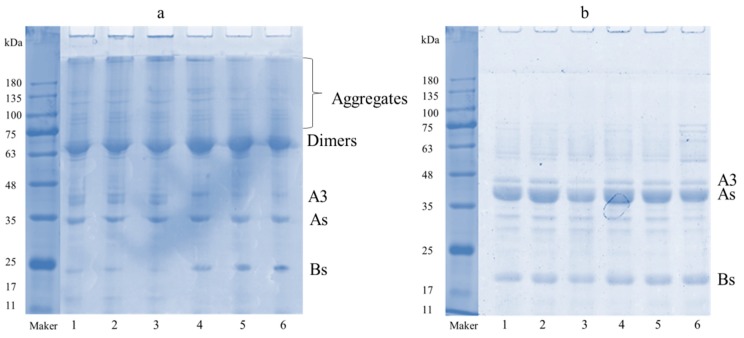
Non-reductive SDS-PAGE electrophoresis profile (a): 1–6 without 2-mercaptoethanol (2-ME) and SDS-PAGE electrophoresis profile (b): 1–6 with 2-ME. 1-Control, 2–50 °C, 3–60 °C, 4-Ultrasound, 5-Ultrasound-50 °C, 6-Ultrasound-60 °C.
